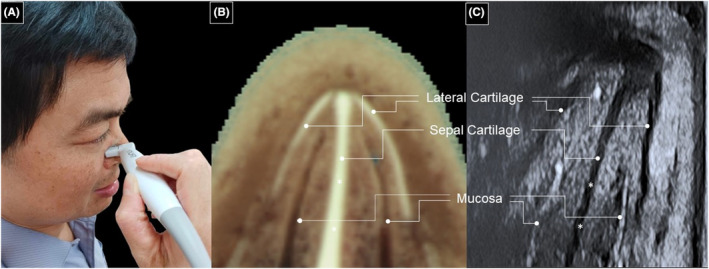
(A) The hockey‐stick transducer is rotated over the lateral wall of the nose, and pressure is exerted from the transducer's footprint to collapse the nasal vestibule. (B) The cadaveric cross‐sectional image and (C) the corresponding ultrasound scan show the nasal cartilage. Note that the bottom part of the nasal cartilage (*asterisk*) is clearly visible in this maneuver. Cadaveric images are adapted from the Visible Human Project of the National Library of Medicine. Excerpts from these images, featured in the VH Dissector, are used with permission from Touch of Life Technologies Inc. Images showing the transducer position on our corresponding author's face are also used with permission for publication.
